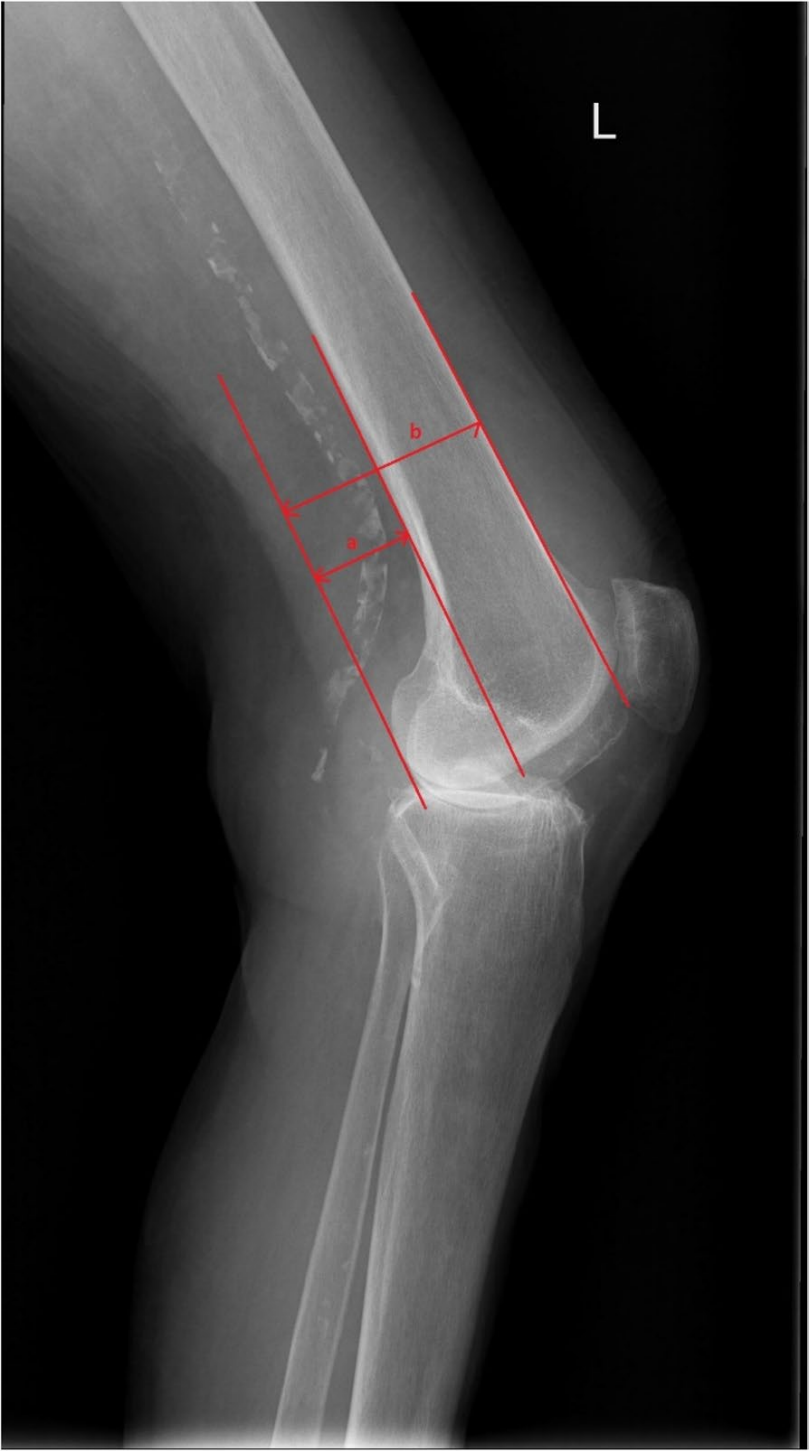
Measurement of preoperative posterior condylar offset ratio (a/b) on standard lateral x-ray
